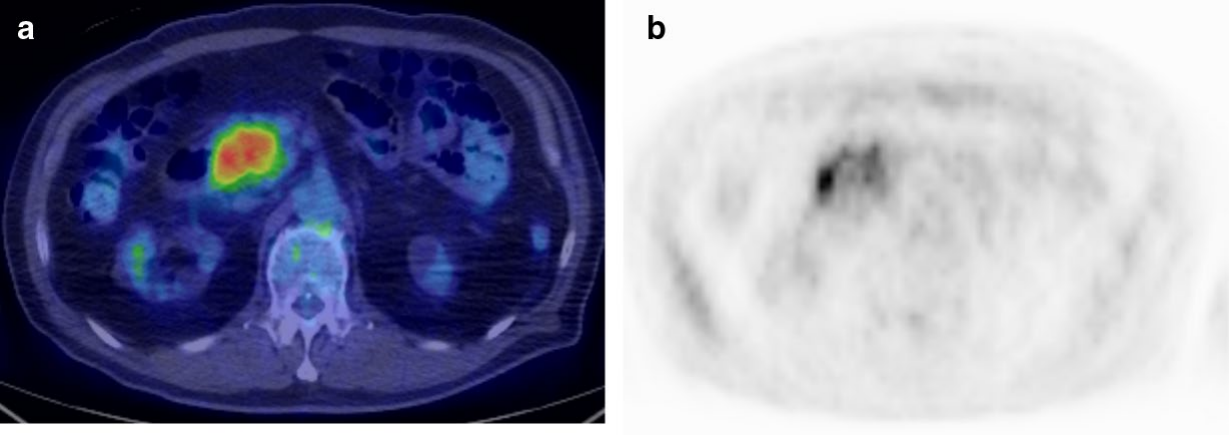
PET/CT. a PET/CT showed positive accumulation of SUVmax 6.02 at the lesion site; b the highest accumulation of about SUV 6.0 was observed near the duodenal side of the mass, and the lowest one at about SUV 3.0 was observed on the opposite side
